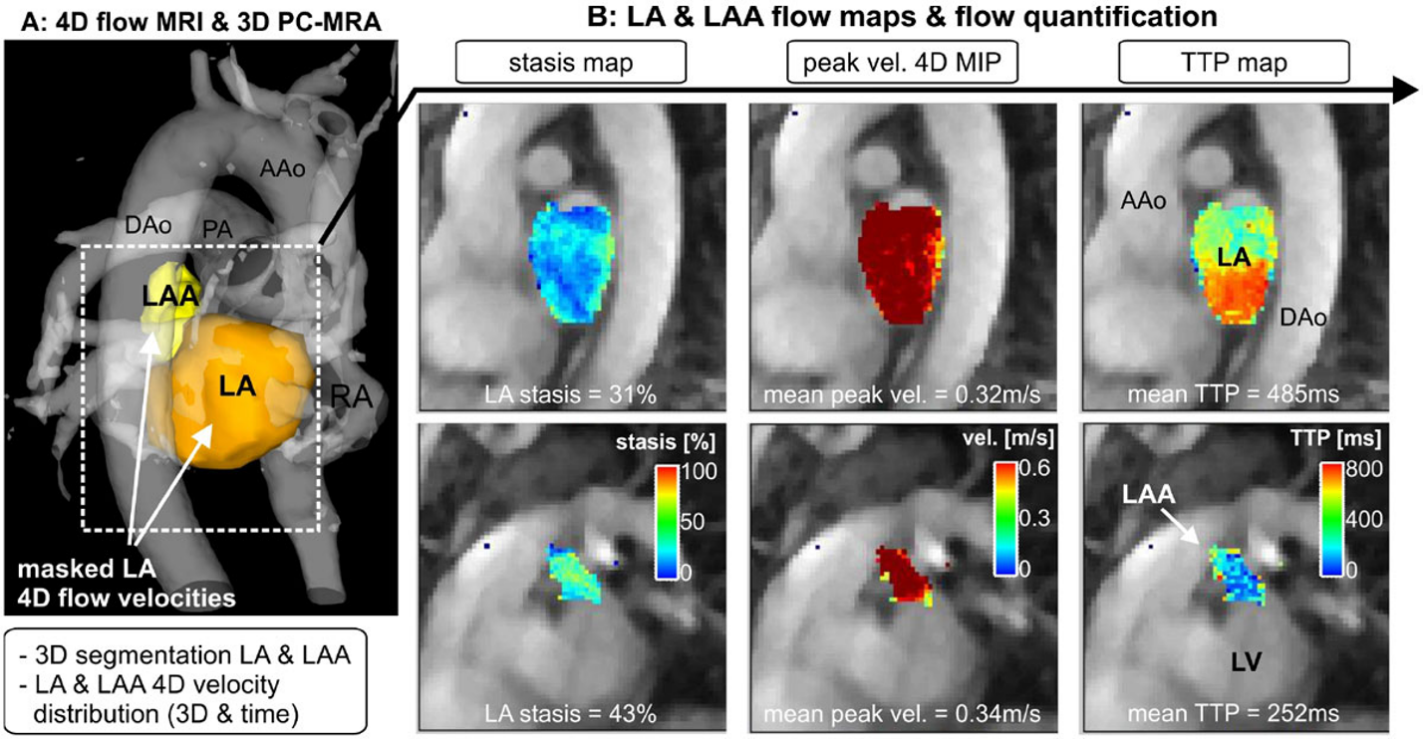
4D flow MRI (A) and derived 3D PC angiogram (PC-MRA) in a male AF patient (EF = 57%, heart rate = 46 bpm, CHA_2_DS_2_-VASc score = 1, in sinus rhythm during the MRI scan). 3D segmentation of the LA and LAA was used to mask the measured time-resolved 3-directional blood flow velocities inside the LA and LAA boundaries for the calculation of LA and LAA stasis, peak velocity and time-to-peak (TTP) maps. (B)AAo/Dao: ascending/descending aorta, RA: right atrium, LV: left ventricle, PA: pulmonary artery.
